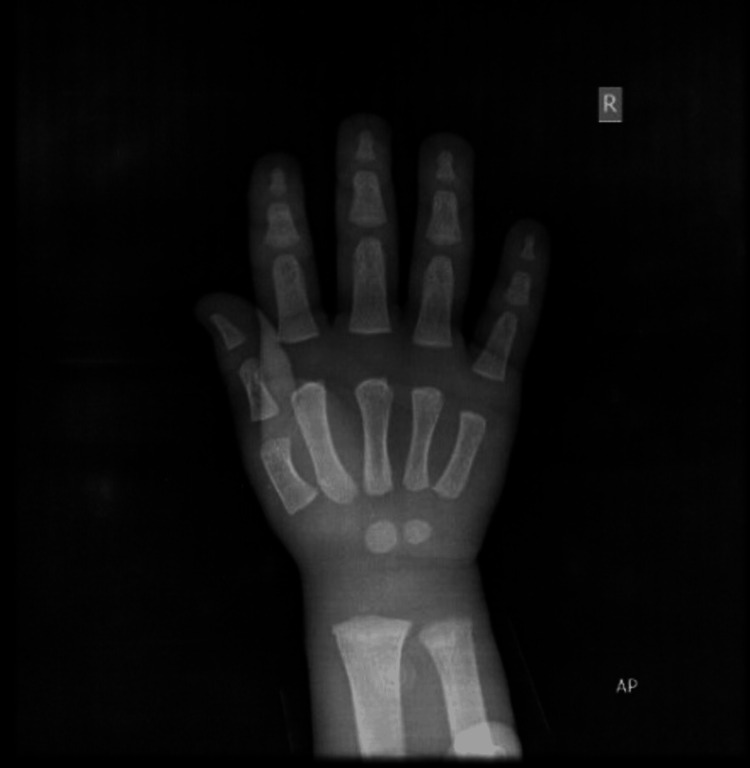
X-ray of the right wrist and hand, which showed a bone age corresponding to the chronological age of one year.
